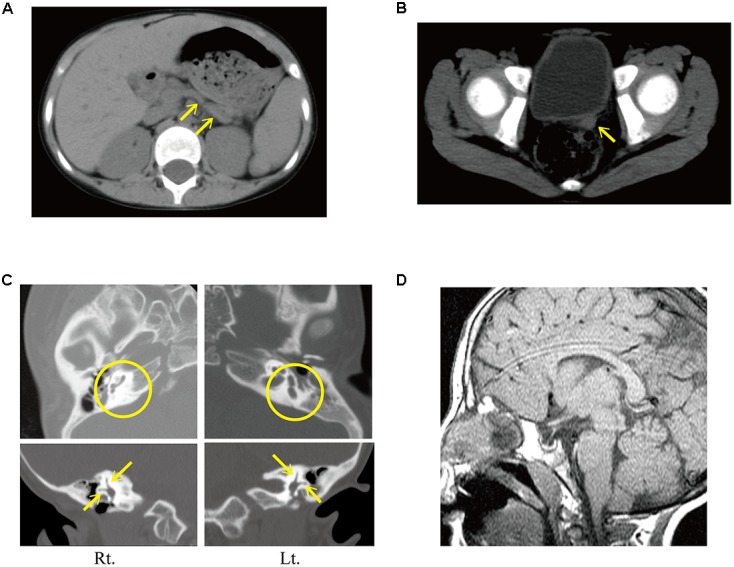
(A–C) Computed tomography findings at age 11 years. (A) Hypoplasia of the pancreatic body and tail (yellow arrow). (B) Uterine hypoplasia (yellow arrow) was identified before estrogen replacement therapy. (C) Computed tomography finding of the internal ear. (Upper images) Aplasia of both posterior semicircular canals (yellow circles). (Lower images) The presence of anterior and lateral semicircular canals was confirmed (yellow arrows). (D) Magnetic resonance imaging showing empty sella.
